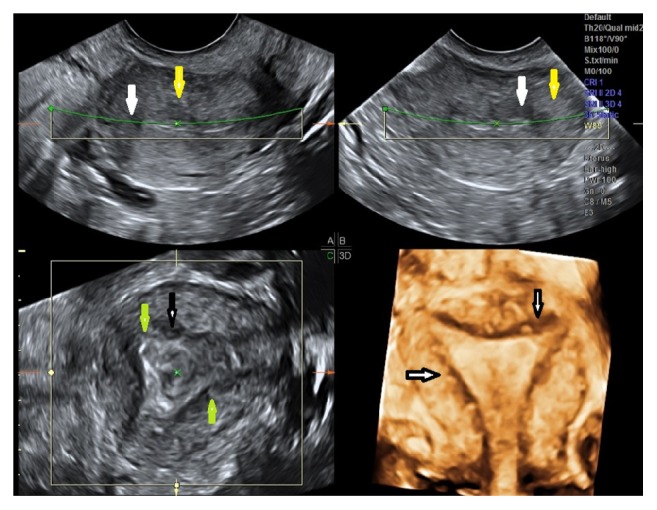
Severe adenomyosis with multiple sonographic signs: multiplanar and 3D rendering of an anteverted uterus with multiple sonographic signs: myometrial cysts (white arrow), hyperechoic islands (yellow arrow), linear striations (green arrow), and irregular EMJ (black arrow).
